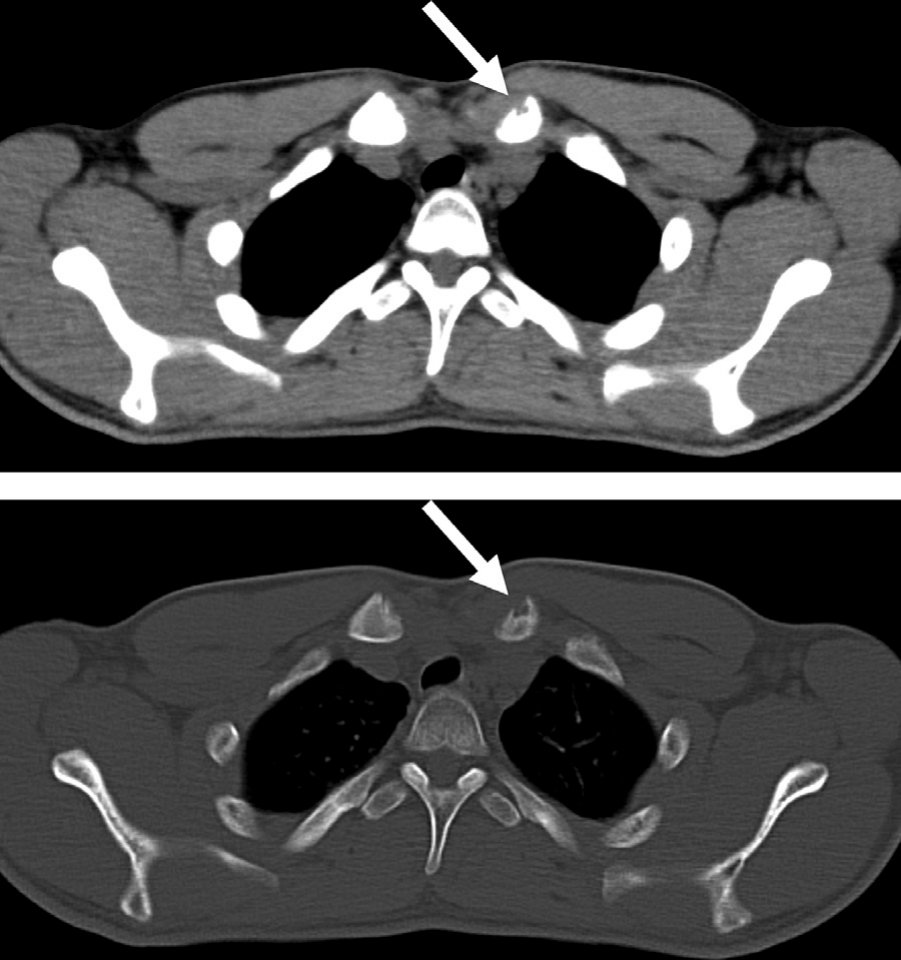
Computed tomography scan showing small osteolytic changes in the left clavicle, consistent with SAPHO syndrome.
